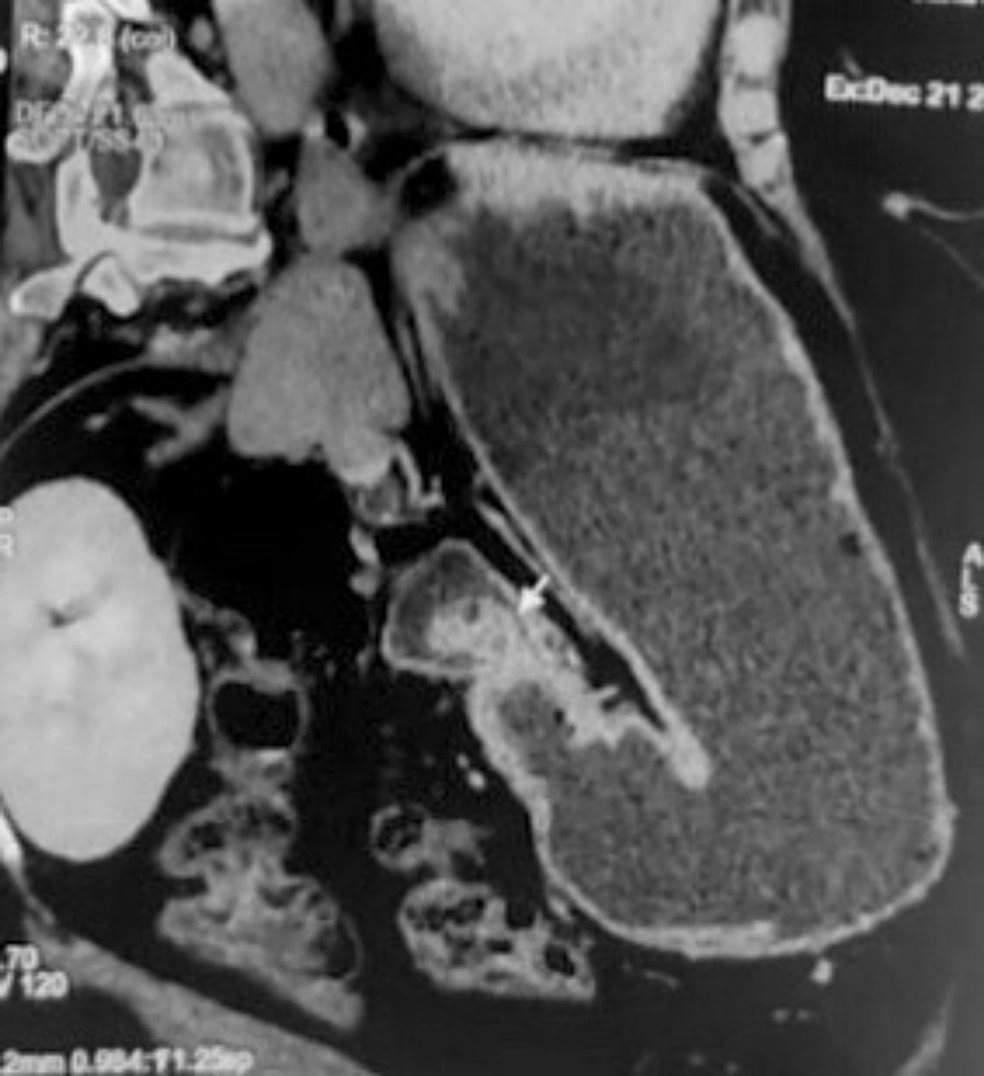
Contrast-enhanced computed tomography showing a homogeneously enhancing polypoidal mass arising from the posterior wall of duodenal bulb measuring 2.3 x 1.5 x 1.5 cm with no periduodenal fat stranding
